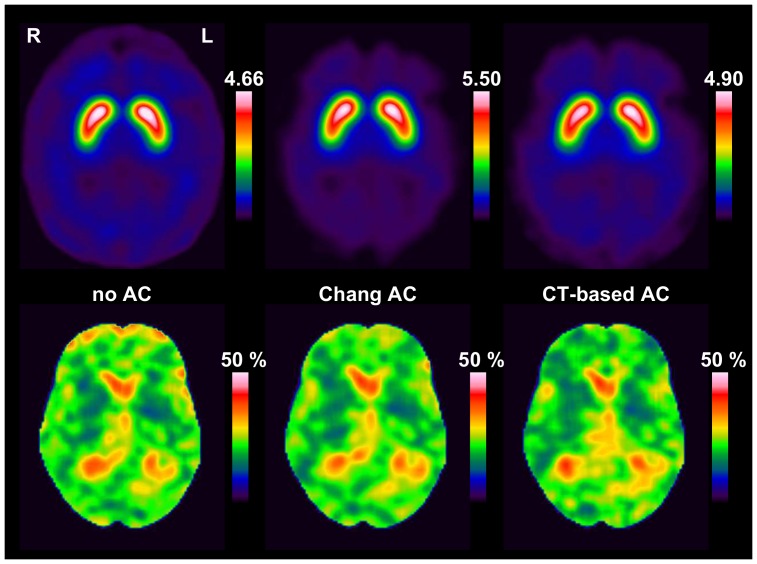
Image quality. Slab of 12 mm thickness of the scaled, stereotactically normalized I-123-ioflupane SPECT averaged over all patients with normal DAT availability (top). Slab displaying the coefficient of variation (%) of the DVR over all patients with normal DAT availability (bottom).
